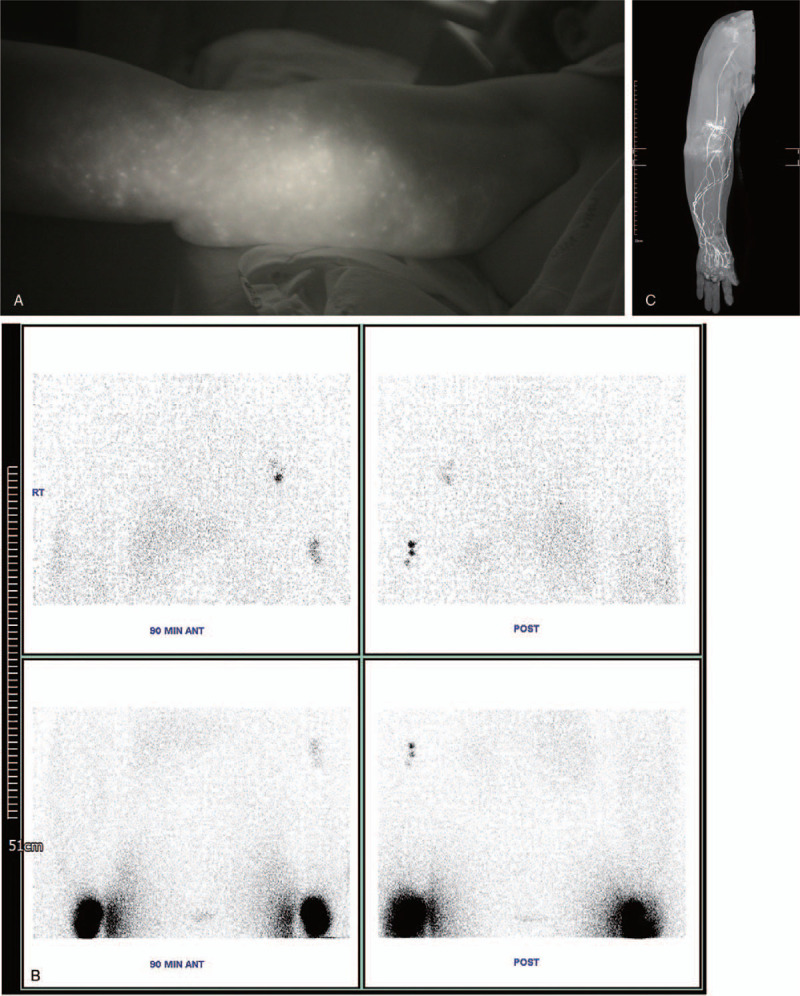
A patient with right upper extremity lymphedema and carpal tunnel syndrome. (A) Indocyanine green lymphography. (B) Lymphoscintigraphy. (C) Magnetic resonance lymphangiography. This patient had International Society of Lymphology late stage 2 lymphedema with confluent dermal backflow and patent lymphatic vessels were visualized.
